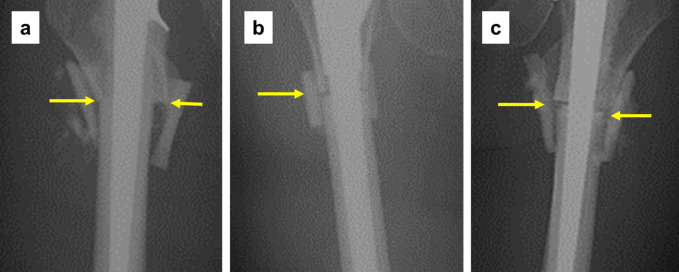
Radiographs showing three types of osteotomy site: A, without a noticeable gap, (B) with a gap of more than 1 mm, and (C) with cement interposition within the gap at the osteotomy site. Arrows indicate osteotomy sites.
